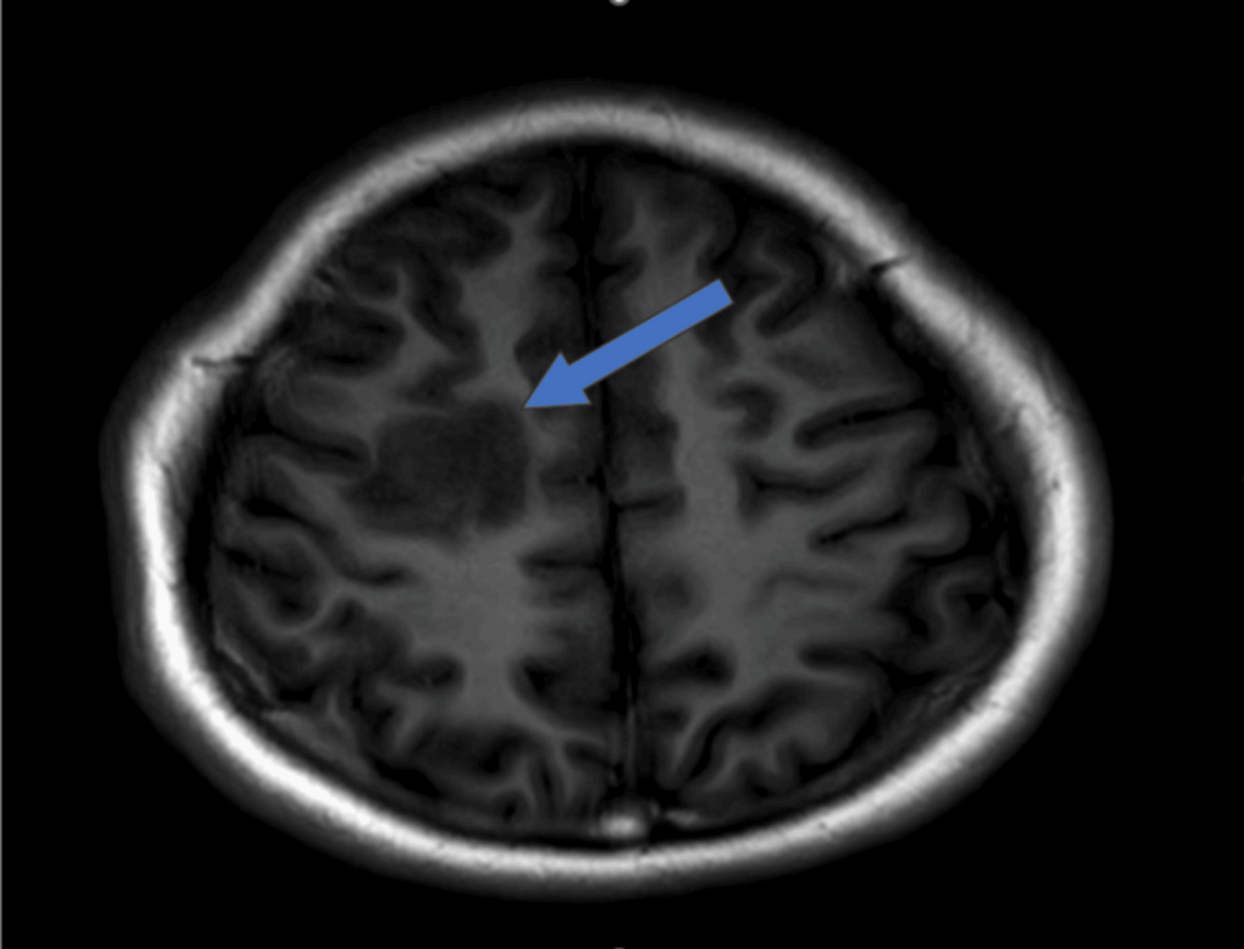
Non-contrast axial T1-weighted MRI image showing a heterogeneous hypointense lesion in the right high frontal lobe at the gray-white matter junction (arrow).
